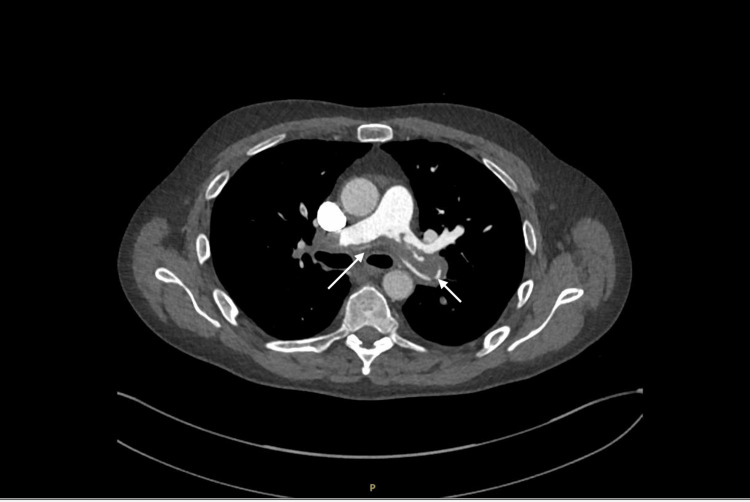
CTPA showing a saddle-shaped embolus (white arrows) CTPA: Computed tomography pulmonary angiography
